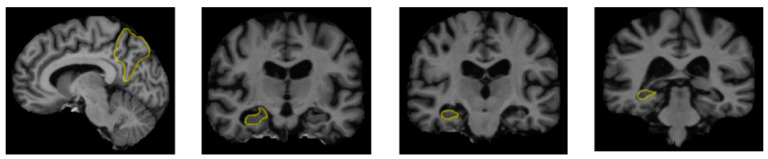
The regions of the brain affected by Alzheimer’s disease in MRI images [30].
